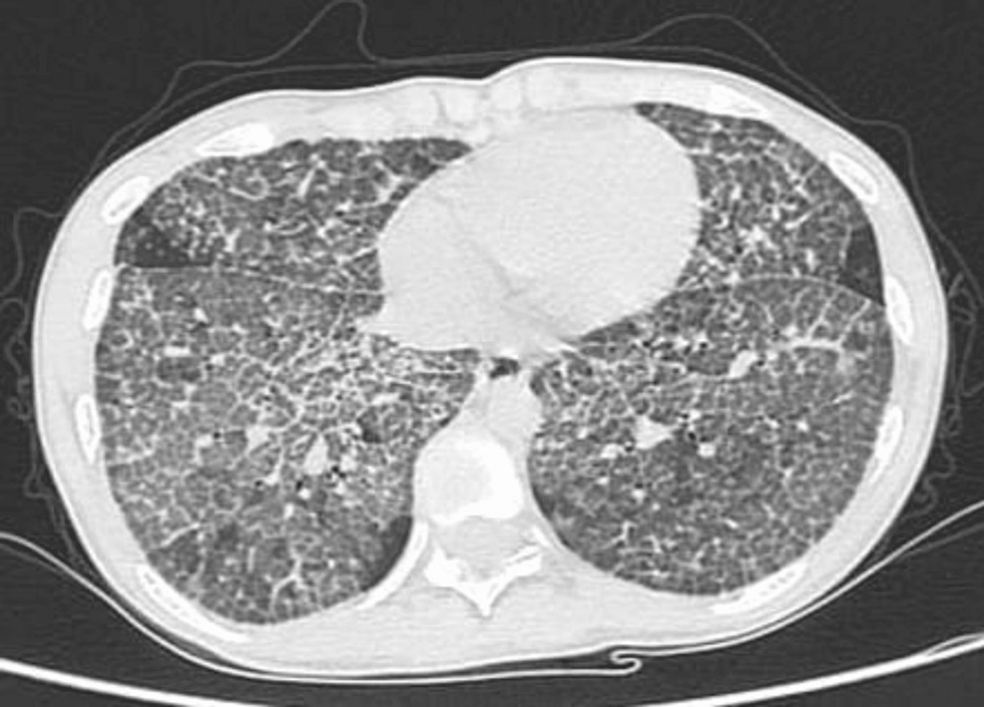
High resolution chest CT scan with crazy-paving appearance.
